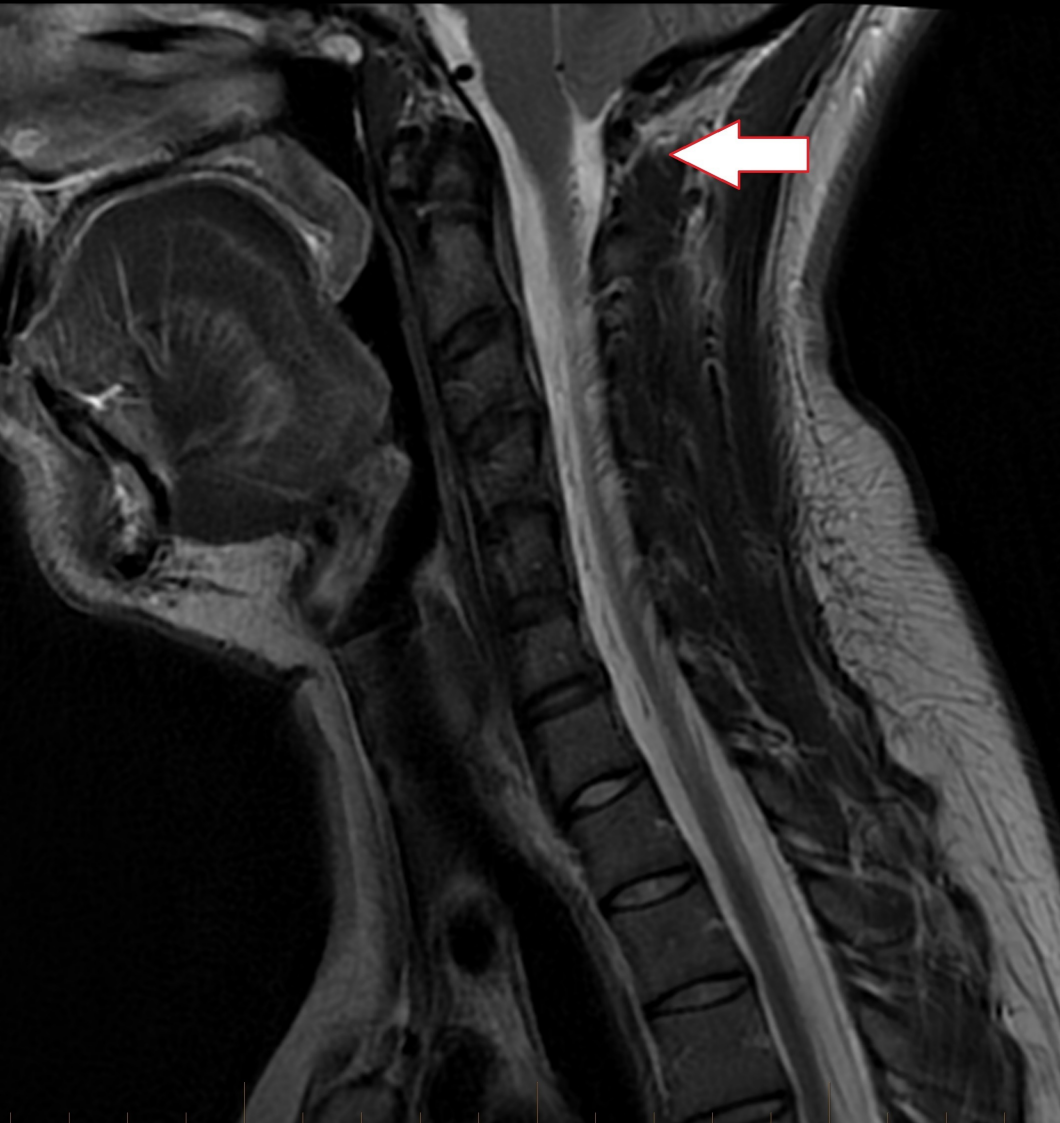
Sagittal T2 cervical spine MRI of mid-line revealing inferior descent of the cerebellar tonsils below the level of the foramen magnum down to the level of the C1 posterior arch
